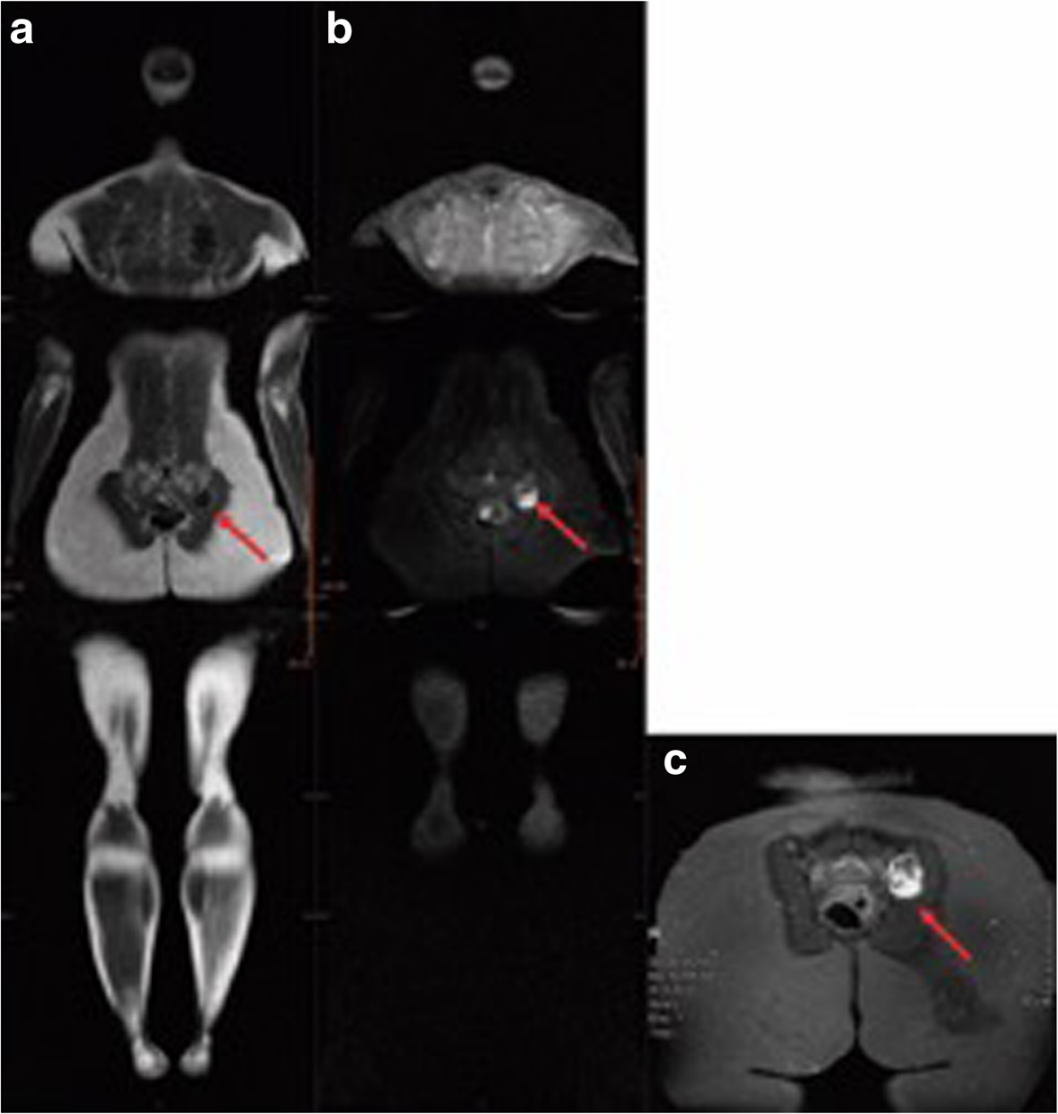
Detection of a sacroiliac joint lesion by WB-MRI and pelvic MRI images of LFS patient, Y0352T000. A T1-weighted image (a) and a STIR image (b) were obtained with WB-MRI. c MRI of the sacroiliac region detected a solid expansive lesion in the posterior-inferior region of the left sacroiliac joint. Bone cortical irregularity was observed in the sacral margin and it was accompanied by bone edema (indicated with arrow)
